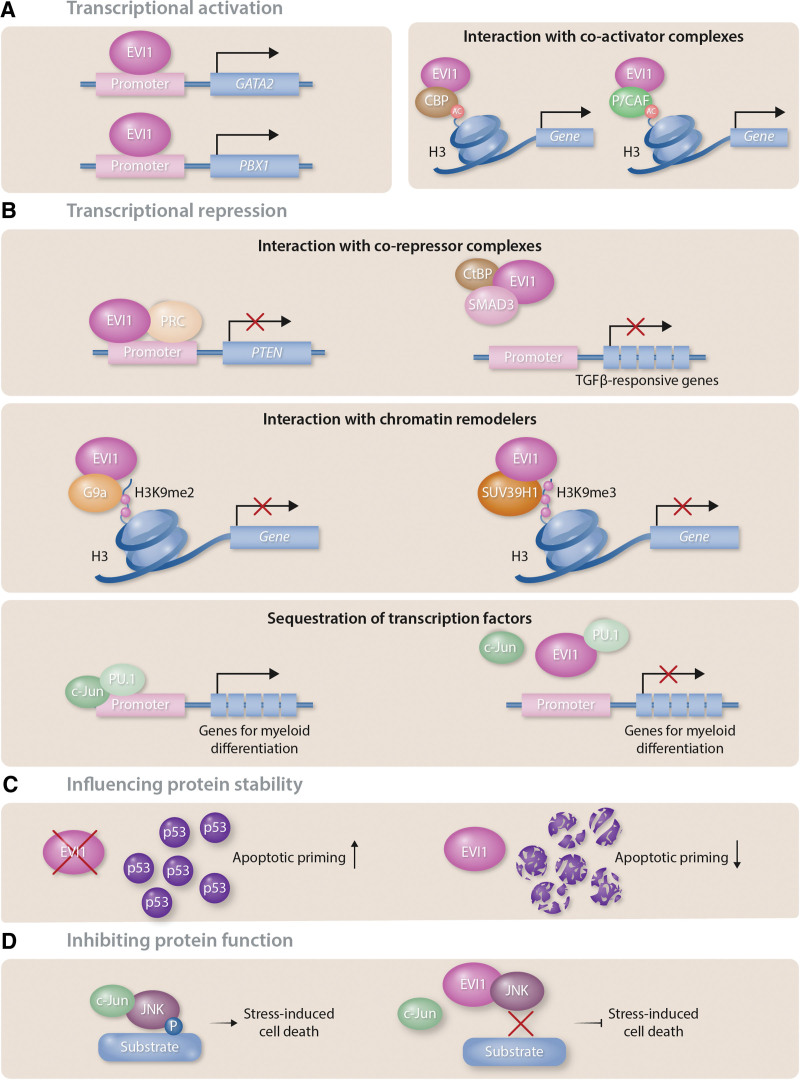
EVI1 protein functions. (A) Transcriptional activation: *Left panel:* EVI1 can act as a transcriptional activator, initiating transcription by directly binding to promoters such as those of *GATA2* and the *PBX1. Right panel:* CBP and P/CAF can interact with EVI1, leading to histone acetylation. (B) Transcriptional repression: *Upper panel:* EVI1 can act as a transcriptional repressor via interaction with corepressor complexes. EVI1 binds both PRC1 and PRC2/3/4 and recruits them to the *PTEN* promoter. EVI1 also forms a co-repressor complex with CtBP and prevents SMAD3 binding to DNA. As a consequence, TGFβ-mediated transcription is inhibited. *Middle panel:* EVI1 interacts with chromatin remodelers such as the histone methyltransferases G9a and SUV39H1, that methylate histone H3 at lysine 9 (H3K9) resulting in transcriptional repression. *Lower panel*: EVI1 binds PU.1 and blocks its interaction with the coactivator c-Jun, thereby preventing PU.1 binding to promoters of genes involved in myeloid differentiation. (C) Influencing protein stability: EVI1 decreases p53 protein abundance via a proteasome-independent mechanism, resulting in decreased apoptotic priming in EVI1^high^ cells. (D) Inhibiting protein function: EVI1 inhibits JNK substrate phosphorylation via direct association with JNK, thus blocking JNK-mediated stress-induced cell death.
